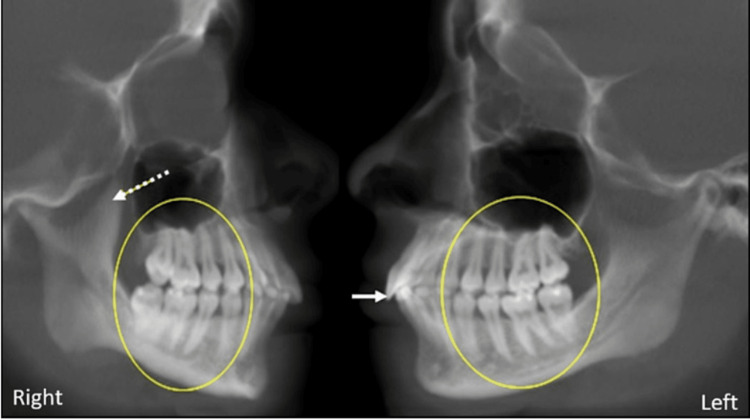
Corrected CBCT sagittal sections at the maximum inter-cuspation position (depicted by yellow circle), Right coronoid hyperplasia (depicted by white dotted arrow), Proclined anterior maxillary teeth (depicted by white solid arrow) CBCT: Cone Beam Computed Tomography
